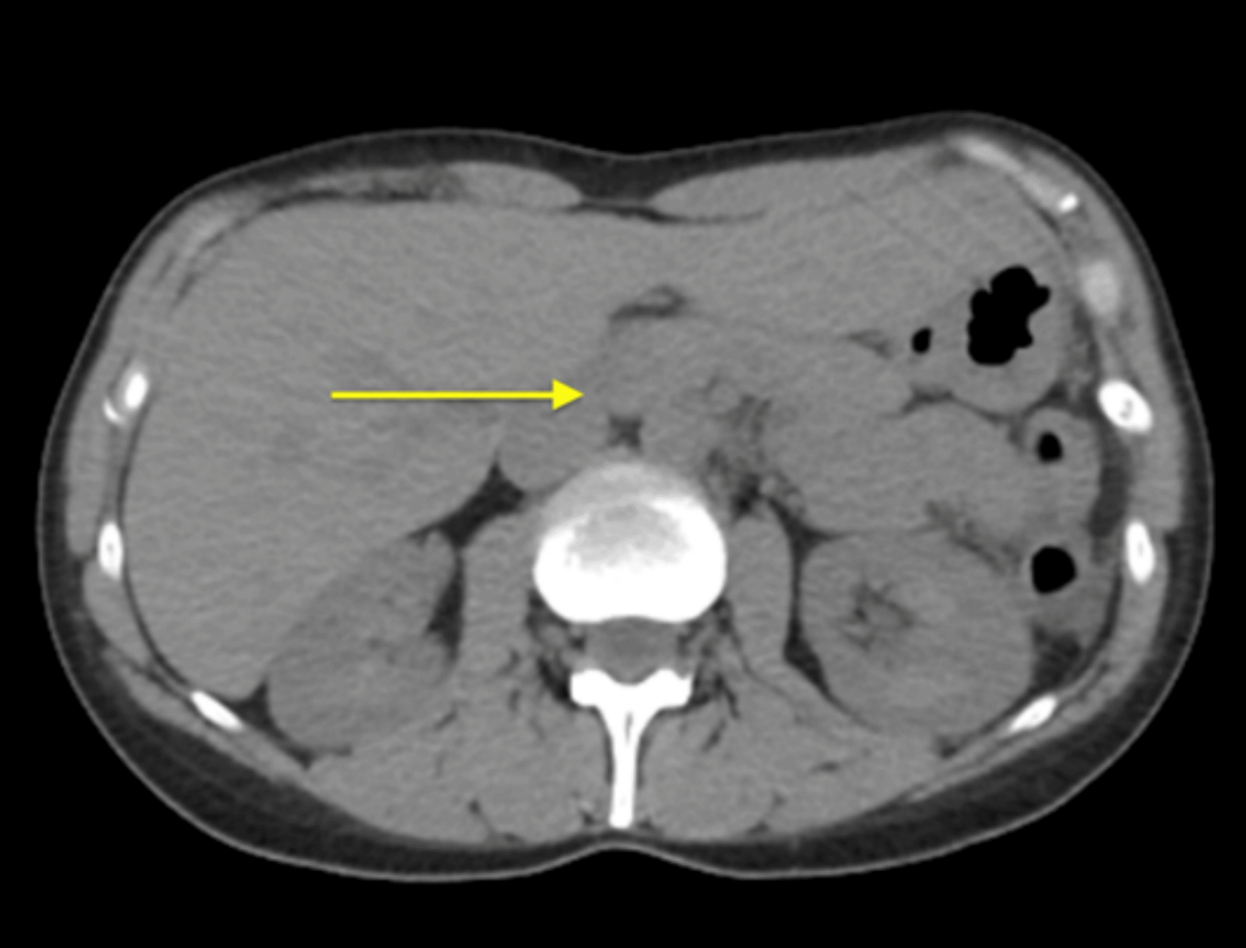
Computerized tomography (CT) abdomen/pelvis without contrast shows mild fat stranding present in the region of the pancreas consistent with acute pancreatitis.
